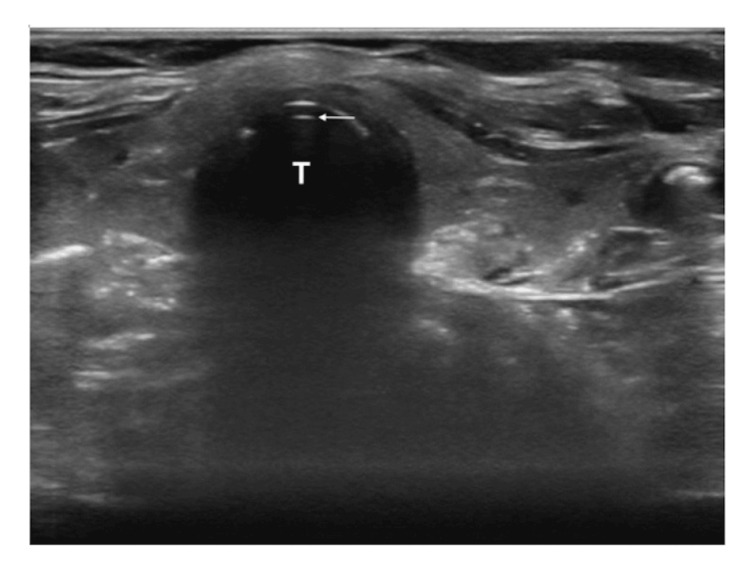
Airway ultrasound showing tracheal intubation The arrow indicates the anterior aspect of the ETT. ETT: endotracheal tube; T: trachea Gottlieb et al. (2024) [35]; Creative Commons Attribution (CC BY) license
